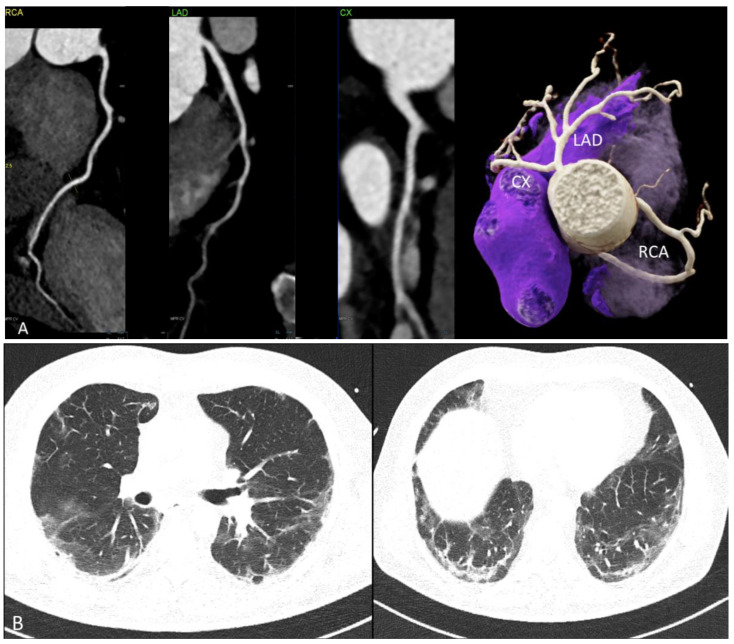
(A) Computed tomography angiography (CTA) showing normal coronary arteries (RCA, LAD and CX). (left: cMPR and right*:* 3D-VRT). (B): Typical lung findings on chest CT: Persisting postinfectious lung injury (PILI) with reticulations and patchy ground glass opacification (GGO).
